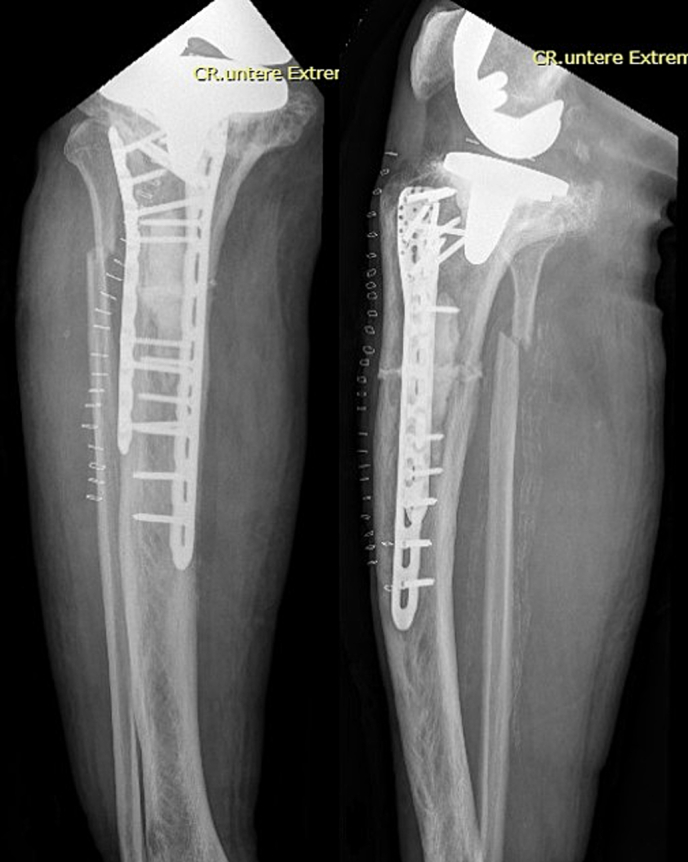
Postoperative AP and lateral radiographs of the right knee and lower leg showing the PHILOS and LCP plates in situ.
